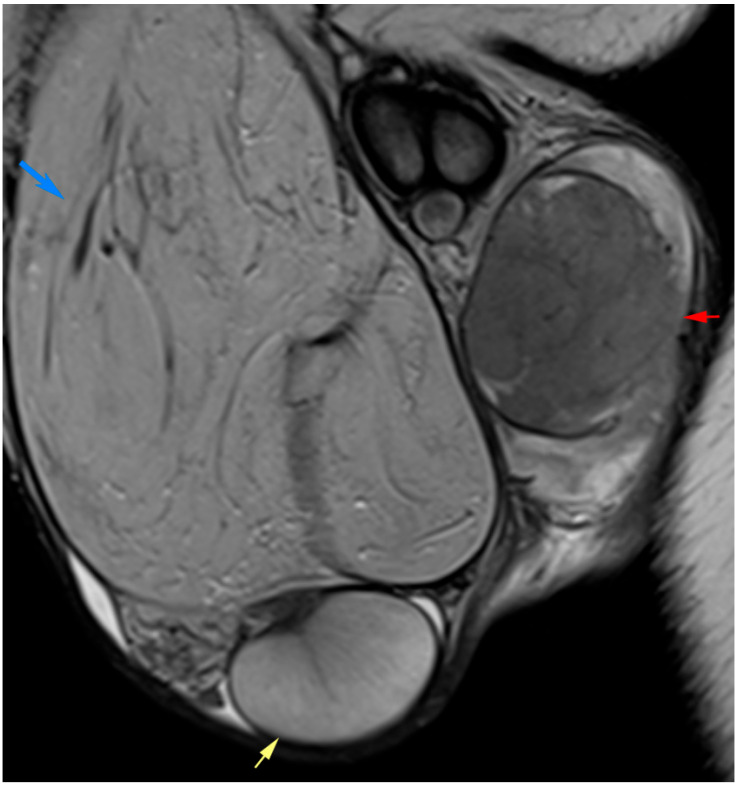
Testicular torsion. Coronal T2-weighted image demonstrates the left testis (red arrow) with irregular margins and markedly hypointense signal consistent with necrosis, in contrast to the normal right testis (yellow arrow). A large right-sided scrotal hernia is also present (blue arrow).
